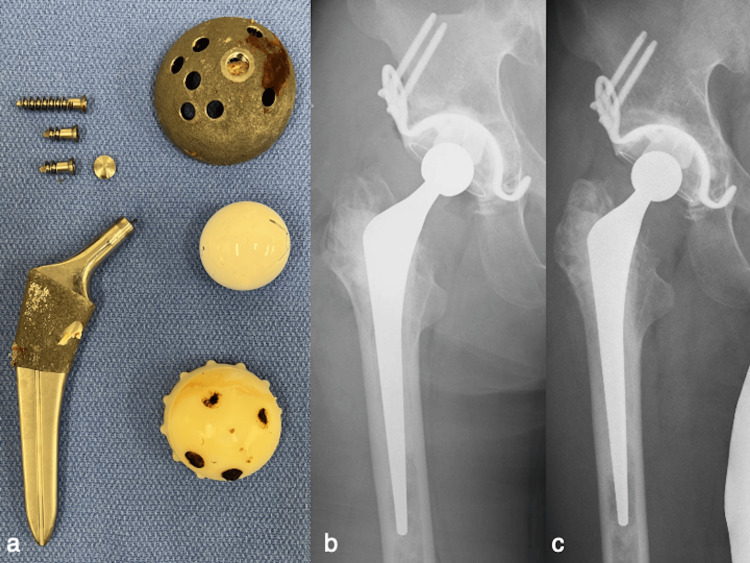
Postoperative findings. (a) Implants show no wear or deformity; (b) the radiograph immediately after the revision; (c) the radiograph obtained one year after the revision shows no recurrent osteolysis.
